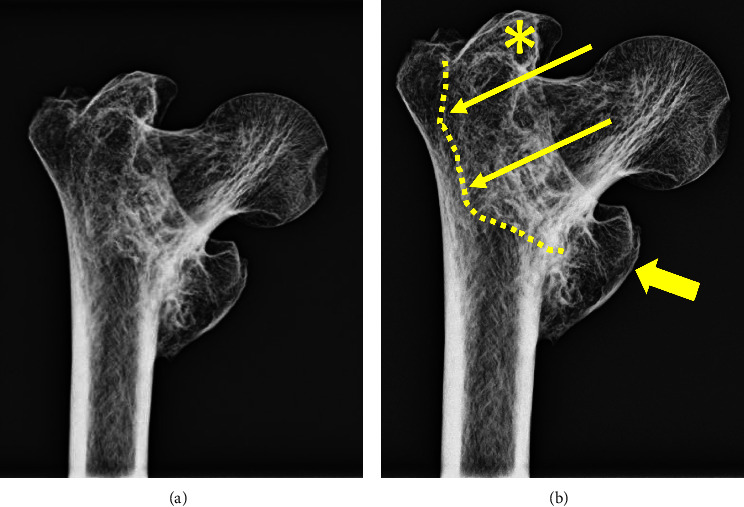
Anteroposterior radiograph of the hip (This is an original figure created by the authors of the manuscript). (a) Without markings. (b) Markings that demonstrate the greater trochanter abducted and medially displaced (yellow asterisk) along with exuberant callus in the region of the lesser trochanter (solid yellow arrow). The faint appearance of an old fracture line can be seen (hatched line and thin yellow arrows).
